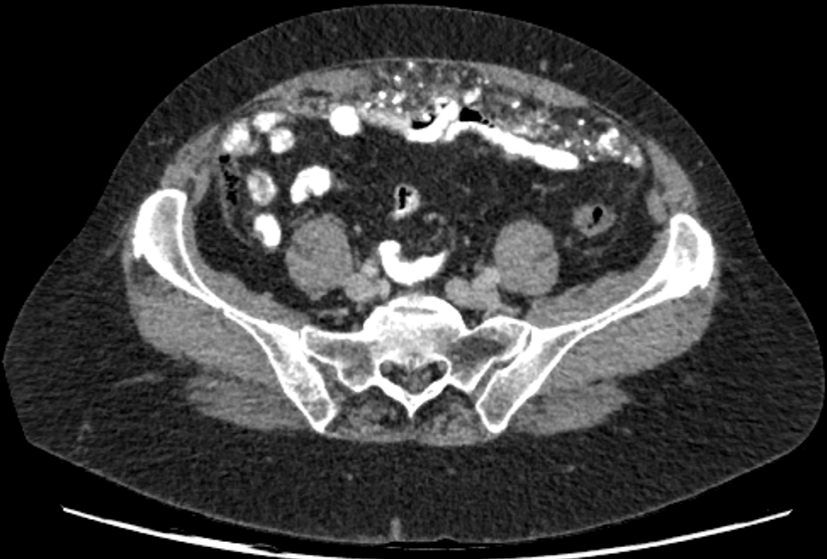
CT axial slice of upper pelvis showing thickening of the omentum which contains a mantle of speckled calcifications.
